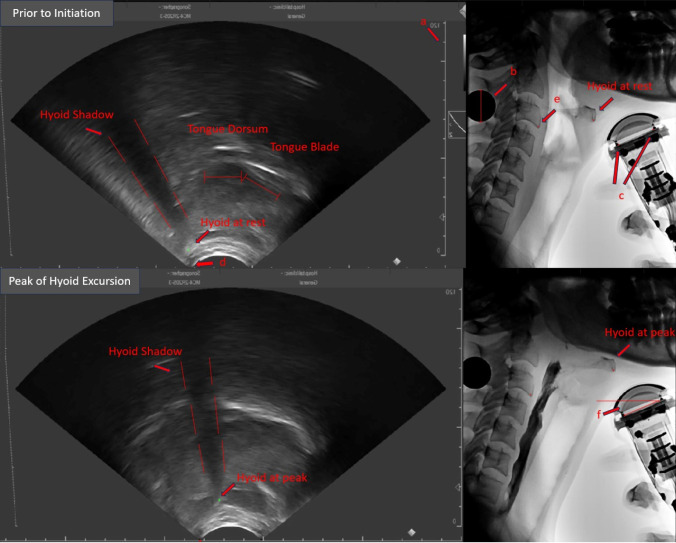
Still frames from ultrasound (left) and MBSS (right), with labeled features and correction components. (A) Ultrasound length calibration scale. (B) MBSS length calibration scale. (C) Internally tracked transducer positions used for calculation of transducer movement. (D) Coordinate reference for correction of ultrasound displacement data. (E) Tracked C2 vertebra used for head movement correction. (F) Angle used to correct ultrasound measurements to MBSS-derived room coordinates
